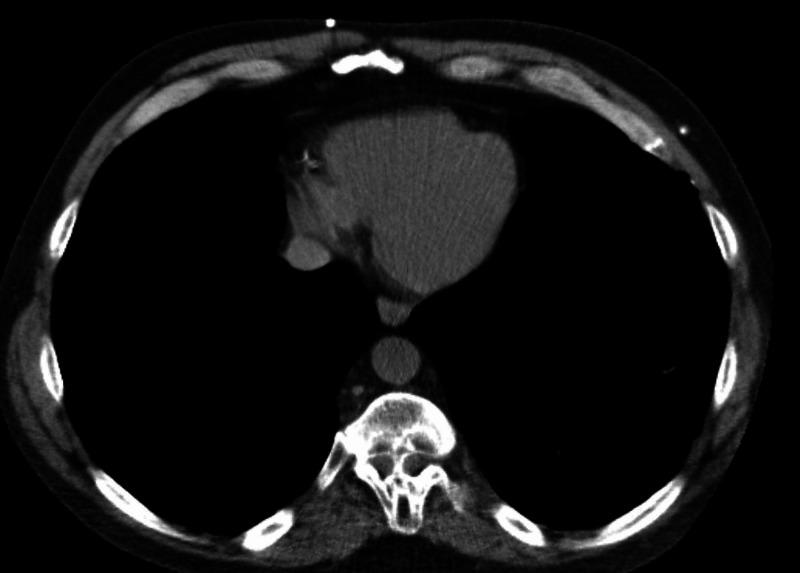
CT of the chest without contrast, axial views. This image demonstrates diastematomyelia of the thoracic spine with a bony septum at approximately T9-T11. There are also partially bifid vertebral bodies at T10 and T11.
